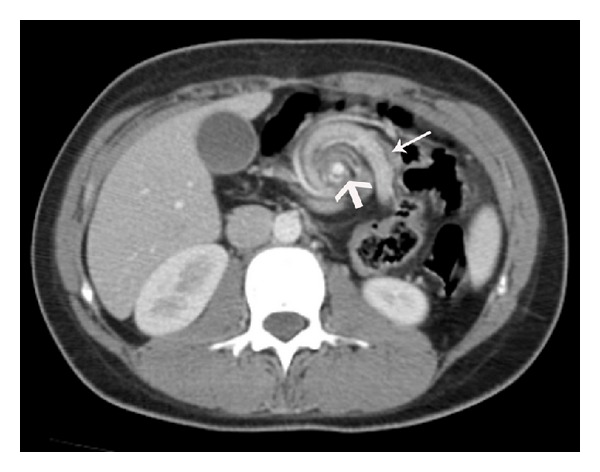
Axial contrast enhanced CT showing characteristic whirl-like appearance of superior mesenteric vein (thin arrow) wrapping around the superior mesenteric artery (thick arrow).
